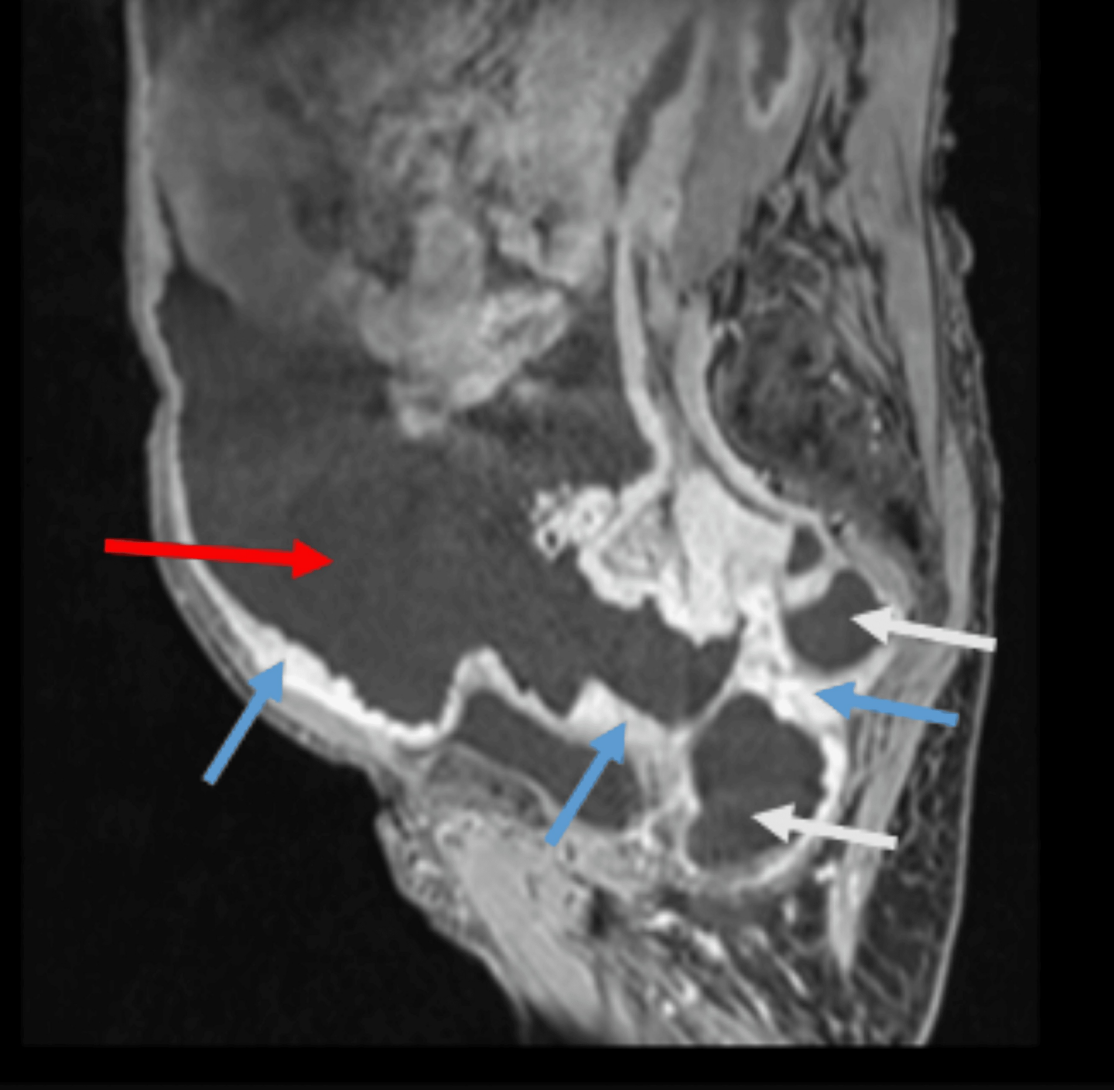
Abdominopelvic MRI revealing characteristic features of peritoneal mesothelioma Coronal MRI image demonstrates large-volume ascites (red arrow) occupying the entire peritoneal cavity. Note the diffuse, nodular peritoneal thickening (blue arrows) and cystic components (white arrows), particularly along the parietal peritoneum. This pattern of diffuse peritoneal involvement, in the absence of a distinct visceral primary tumor, is highly suggestive of peritoneal mesothelioma and helps distinguish it from localized gastrointestinal cancers or the more focal spread of some ovarian malignancies.
